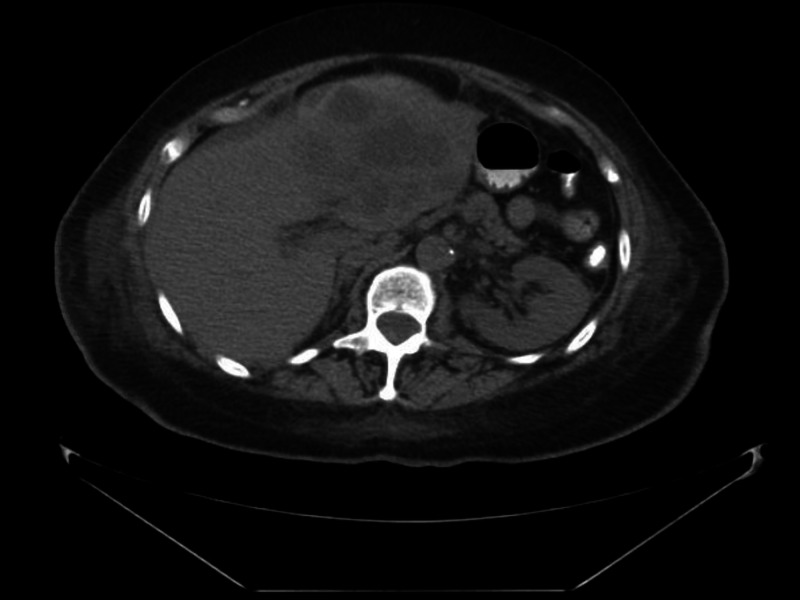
Axial CT images of the abdomen and pelvis w/o contrast demonstrating a solid mass within the left lobe involving segments II, III, and IV measuring 6.7 x 7.8 x 6.5 cm, which could represent primary or metastatic tumor CT: computed tomography
